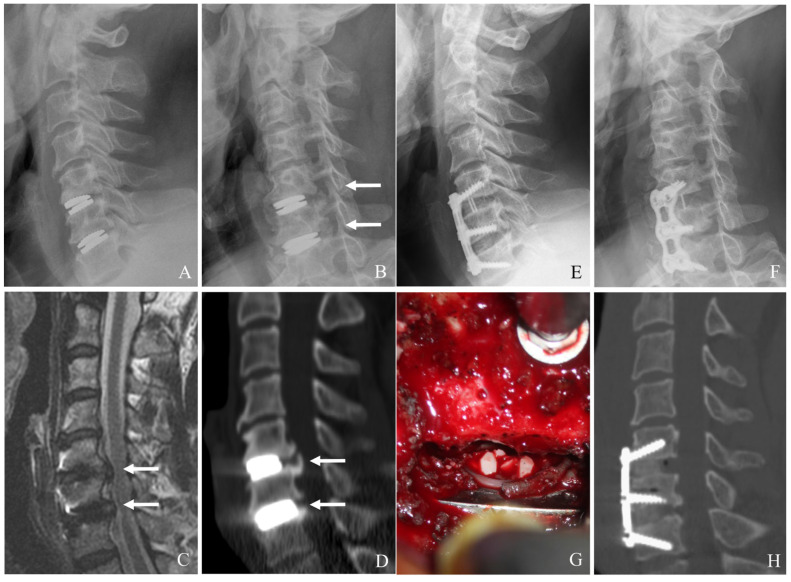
Plain X-rays (A,B), magnetic resonance image (C), and computed tomography (D) showing cervical disc arthroplasty at C5–6 and C6–7 with severe foraminal stenosis and posterior osteophytes (white arrows). The patient underwent removal of C5–6 and C6–7 prosthesis and revisional anterior cervical discectomy and fusion (E–H).
